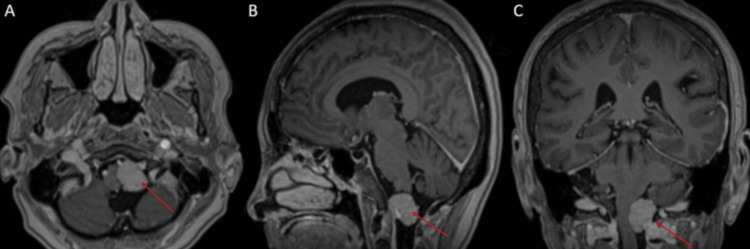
(A) Axial, (B) sagittal, and (C) coronal T1-weighted MRI with gadolinium enhancement revealing a well-defined, homogeneously enhancing mass (red arrow) at the left foramen magnum, measuring 21 x 20 x 23 mm. The mass exerts pressure on the left medulla, causing rightward deviation.
